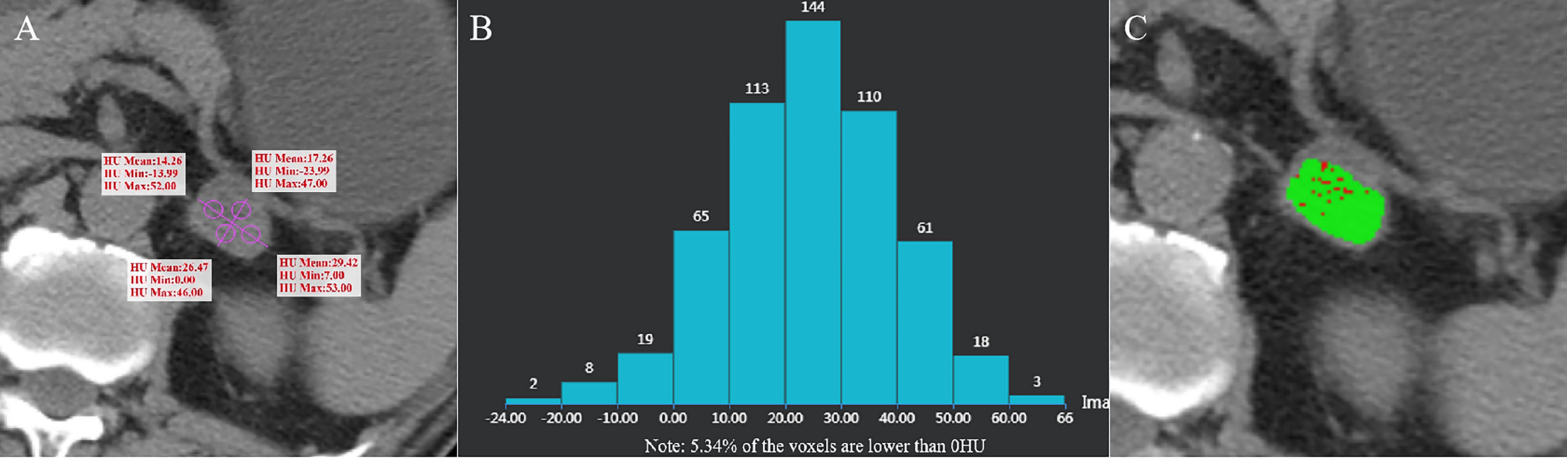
Adrenal adenoma of the left adrenal gland in a 62-year-old man. AV_mean_ =21.853 HU (A), AV_min_ = -7.745 HU, and CTH negative pixel =5.34% (B). The CT value in the red area is ≤ 10 HU, and the CT value in the green area is > 10 HU (C).
